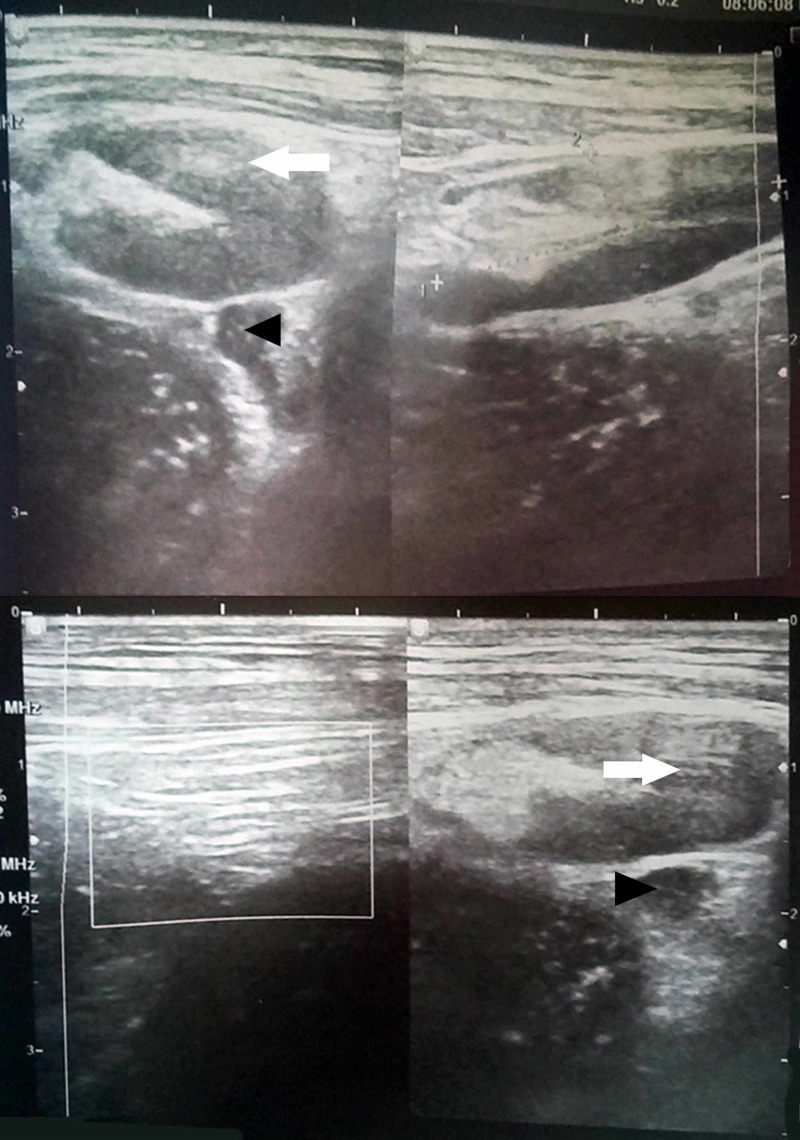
USG showing soft tissue collection (white arrows) and sub-periosteal collection (black arrowheads) over anteriomedial aspect of the right proximal leg suggestive of acute osteomyelitis. USG, ultrasonography
